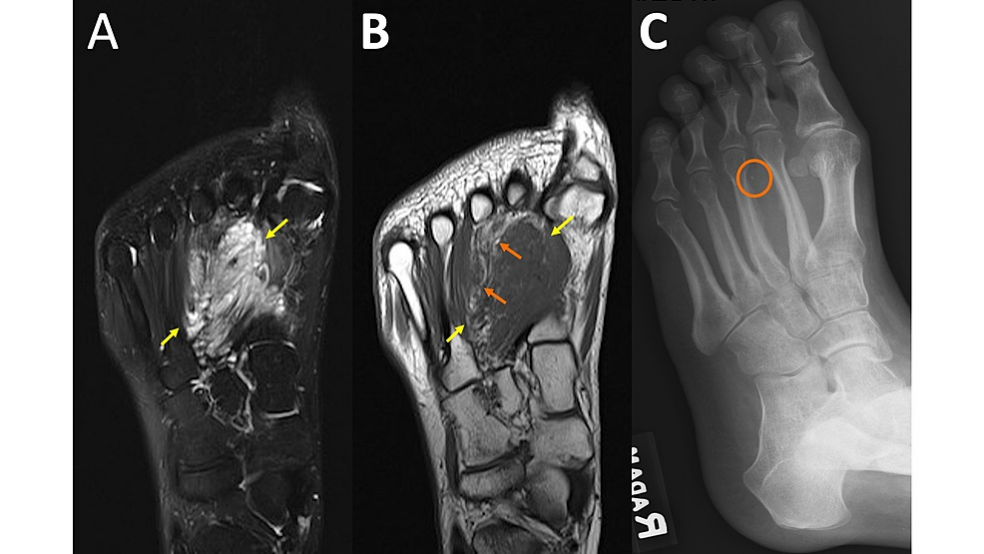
Vascular Malformation MRI long-axis T2 fat saturation (FS) (11A) and MRI long-axis T1 (11B) demonstrate a heterogenous predominately T2 hyperintense, T1 hypointense, lobulated, somewhat infiltrating mass (yellow arrows). There are some areas of T1 hyperintense signal suggesting intralesional fat (orange arrows), which is helpful for forming a differential diagnosis; however, the MRI is nonspecific. Considerations, in this case, would include but are not limited to a Desmond tumor, vascular malformation, or synovial sarcoma. Oblique foot radiograph (11C) demonstrates mild splaying of the second and third metatarsal bones with the chronic remodeling of the third metatarsal diaphysis, and the most important finding, soft tissue calcification consistent with a phlebolith (circle). This key feature helps establish the diagnosis of hemangioma, precluding the need for biopsy.
